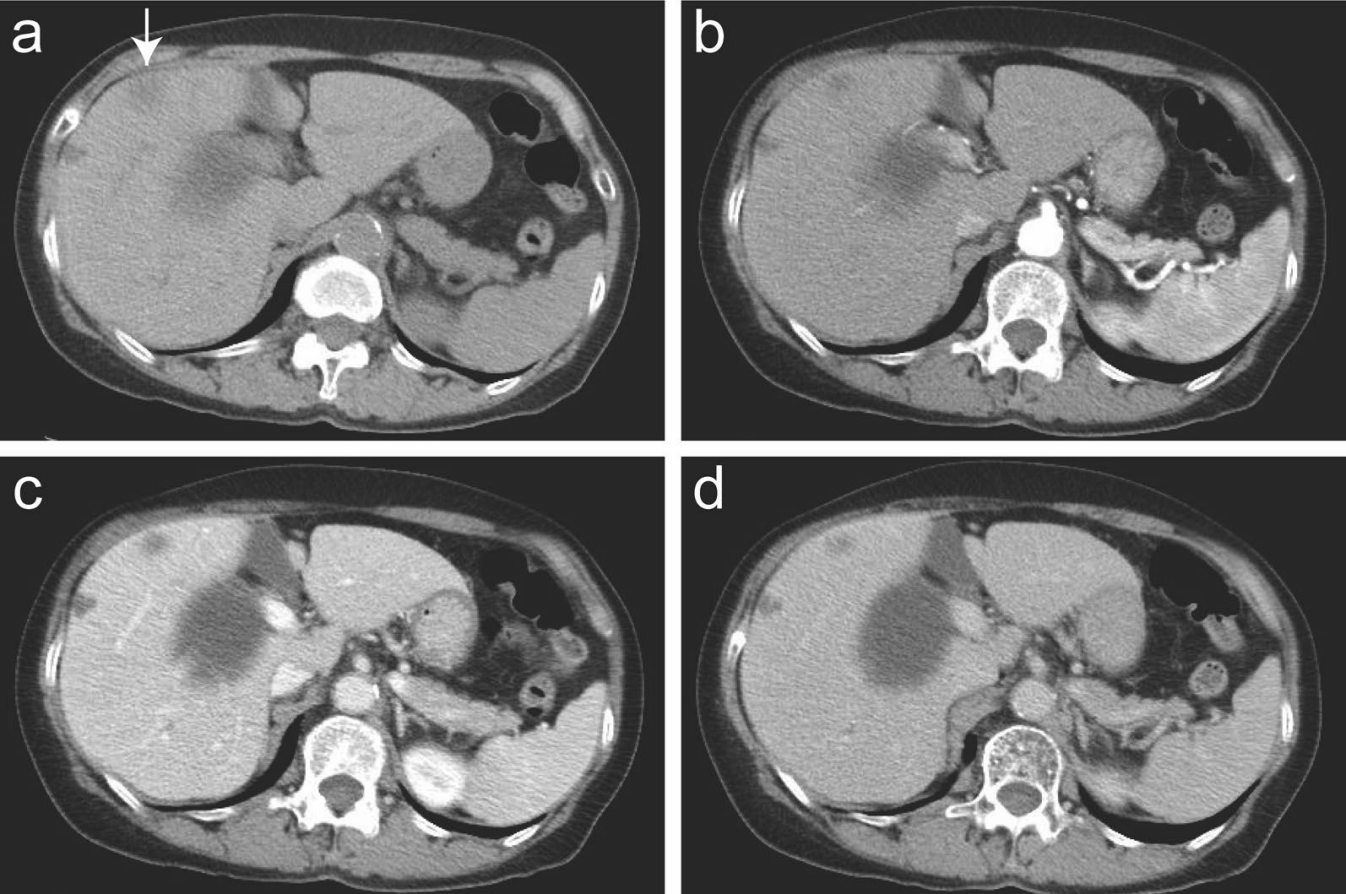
Contrast-enhanced dynamic computed tomography (CT). A plane CT scan showing an ambiguous and low-density mass (2.2 cm in diameter) in liver segment 8 (a). Arterial, portal, and venous phase CT scans showing fair marginal enhancement in all phases (b–d) and fair delayed enhancement in the inner area (d)
